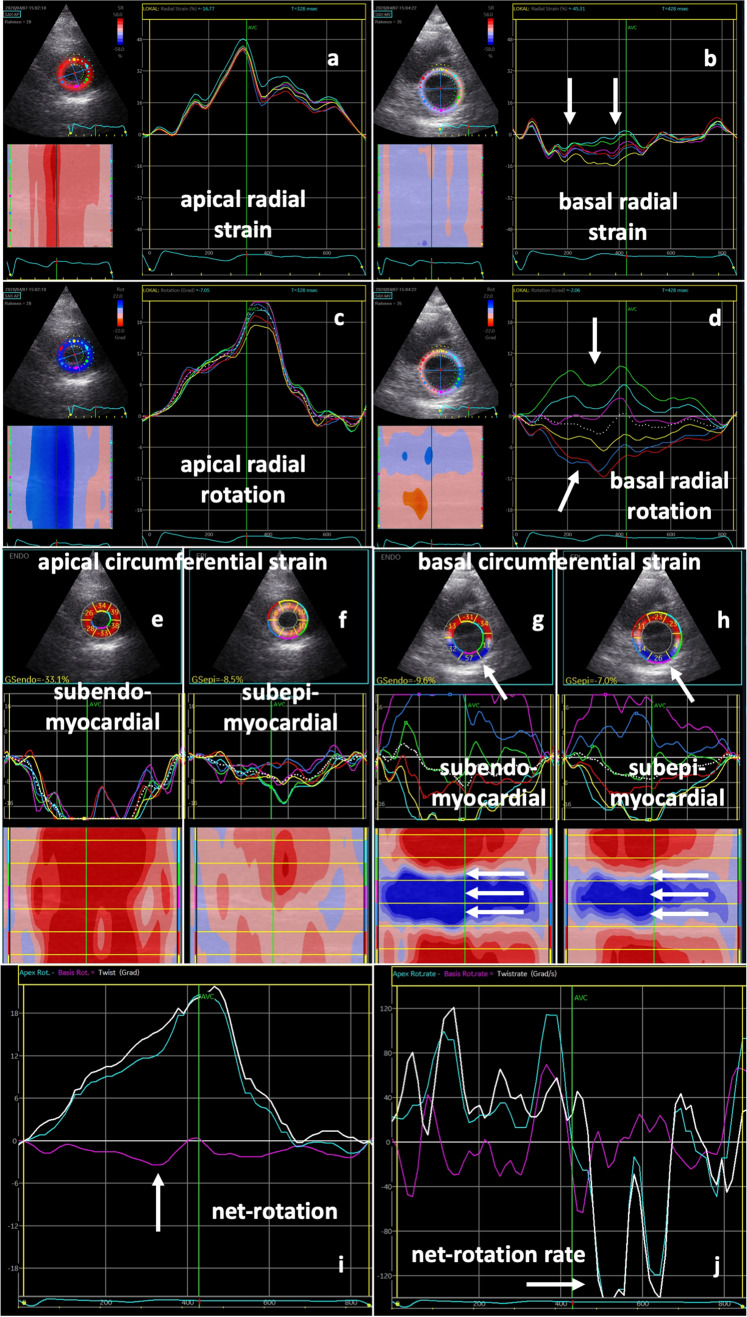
Rotational deformation pattern in another SARS-CoV-2-infected patient with severe symptoms: normal radial strain patterns are documented in apical (a), whereas abnormal patterns are shown in basal (b) left-ventricular (LV) segments. Apical rotation was also normal (c), whereas basal rotation showed a regional dispersion with inverse rotation in various LV segments (d, white arrows). Below parasternal short-axis views with segmental subendomyocardial (e, g) and subepimyocardial (f, h ) circumferential strain values, strain curves, and color-M-Modes are shown. Abnormal deformation is documented basal inferior, inferolateral, and anterolateral (g, h white arrows). Line graphs of apical (blue) and basal rotation (magenta) as well as net rotation (twist) (white) (i) and corresponding line graphs of rotation rate (j) document abnormal basal rotation with compensated twist by pronounced apical rotation (i white arrows) and a “chaotic” pattern of net-rotation rate during diastole (j)
